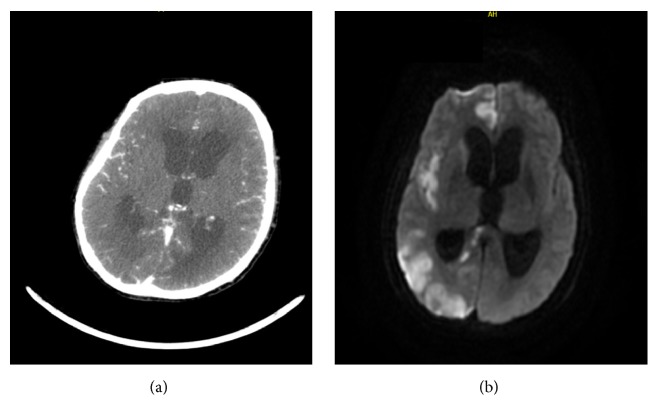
(a) CT angiography of the head showing important supratentorial hydrocephalus with narrowing of cortical sulci and cerebral edema. (b) MRI-scan of the head showing extensive recent ischemia in the cerebellum and dilatation of the third and fourth ventricle.
